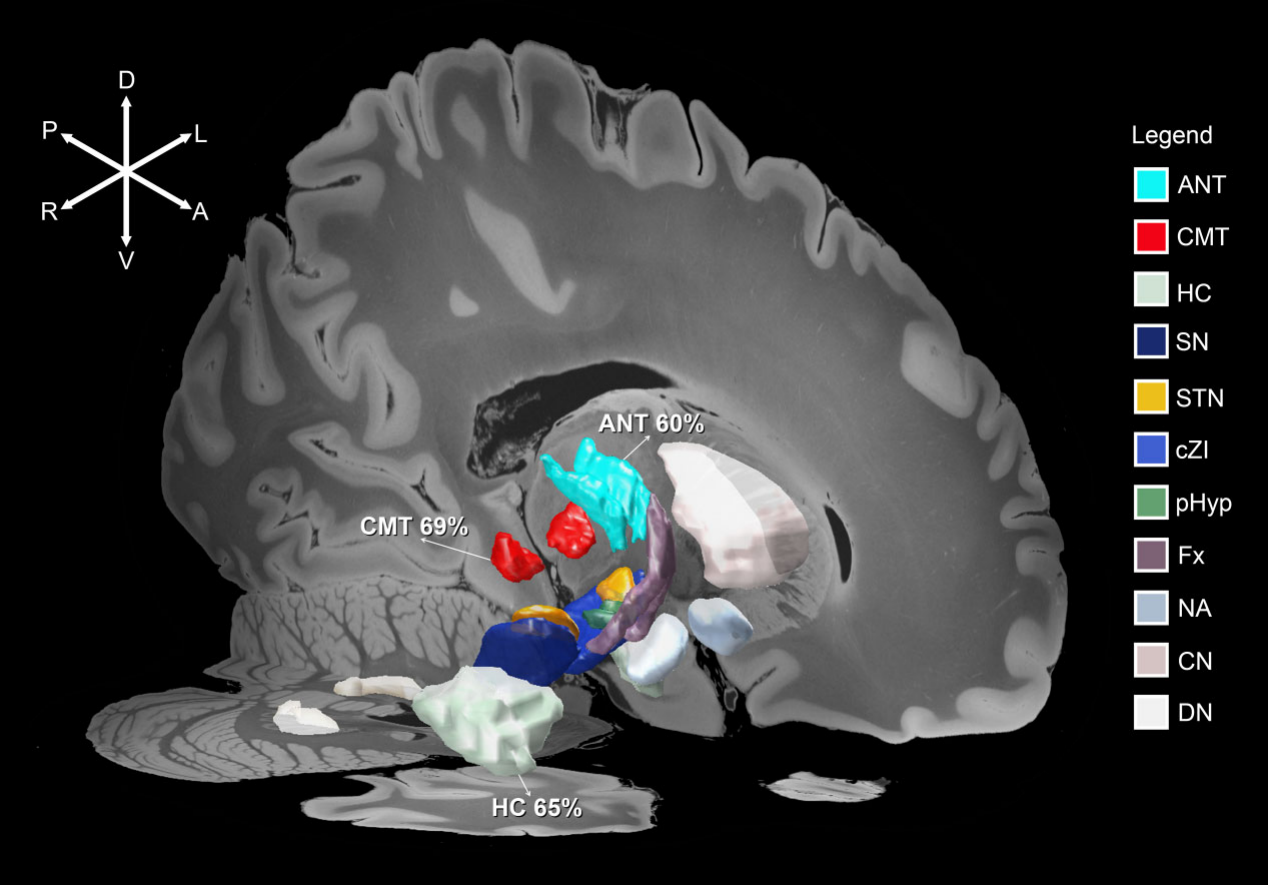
Targets for DBS in epilepsy. 3D representation of anatomical seeds of DBS targets used for the treatment of epilepsy is shown on sagittal and axial 7-Tesla T1 MRI slices of the human brain (100 μm resolution in MNI152 space). Mean seizure reduction that was calculated from our systematic literature review is shown for ANT – 60%, CMT – 69%, and HC – 65%. Based on our systematic review, higher quality of evidence exists for the use of ANT-DBS in DRE. Other DBS targets that belong to known cortico-subcortical circuits are visualized on the figure.
